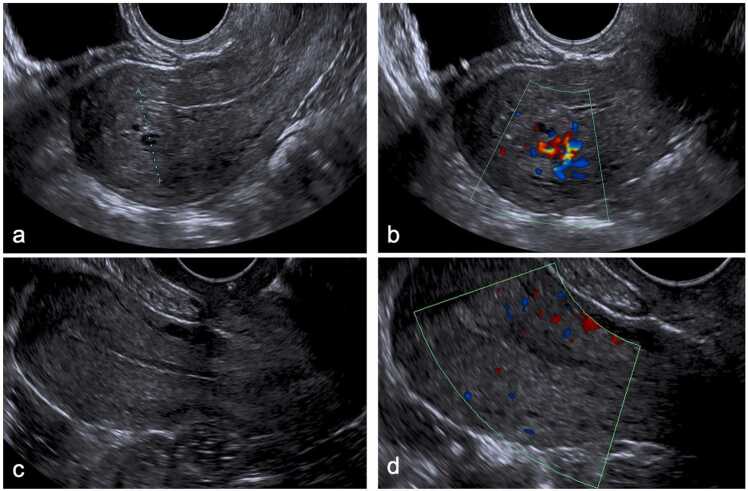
Retained products of conception: a) Ultrasound demonstrates echogenic mass (dotted line) within the endometrial cavity with b) Increased vascularity on Doppler. Post surgical evacuation of products of conception, return to c) Normal endometrial stripe and d) Normal vascularity. DIAGNOSIS: Retained products of conception.
